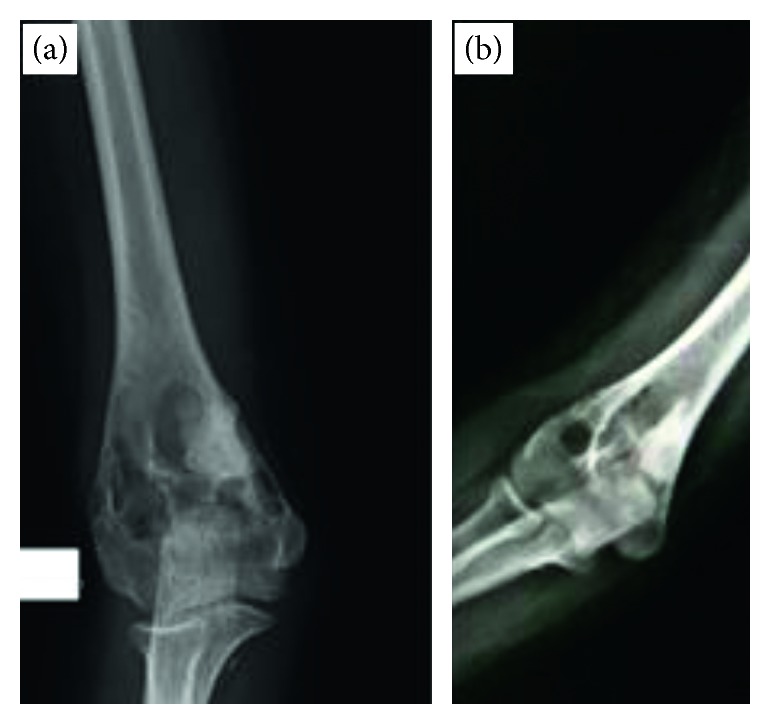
(a) AP X-ray of the distal humerus solid variant ABC about 4 years after surgery showing signs of recurrence. (b) X-ray repeated one year later with no further intervention showing almost complete healing and remodeling of the cyst.
